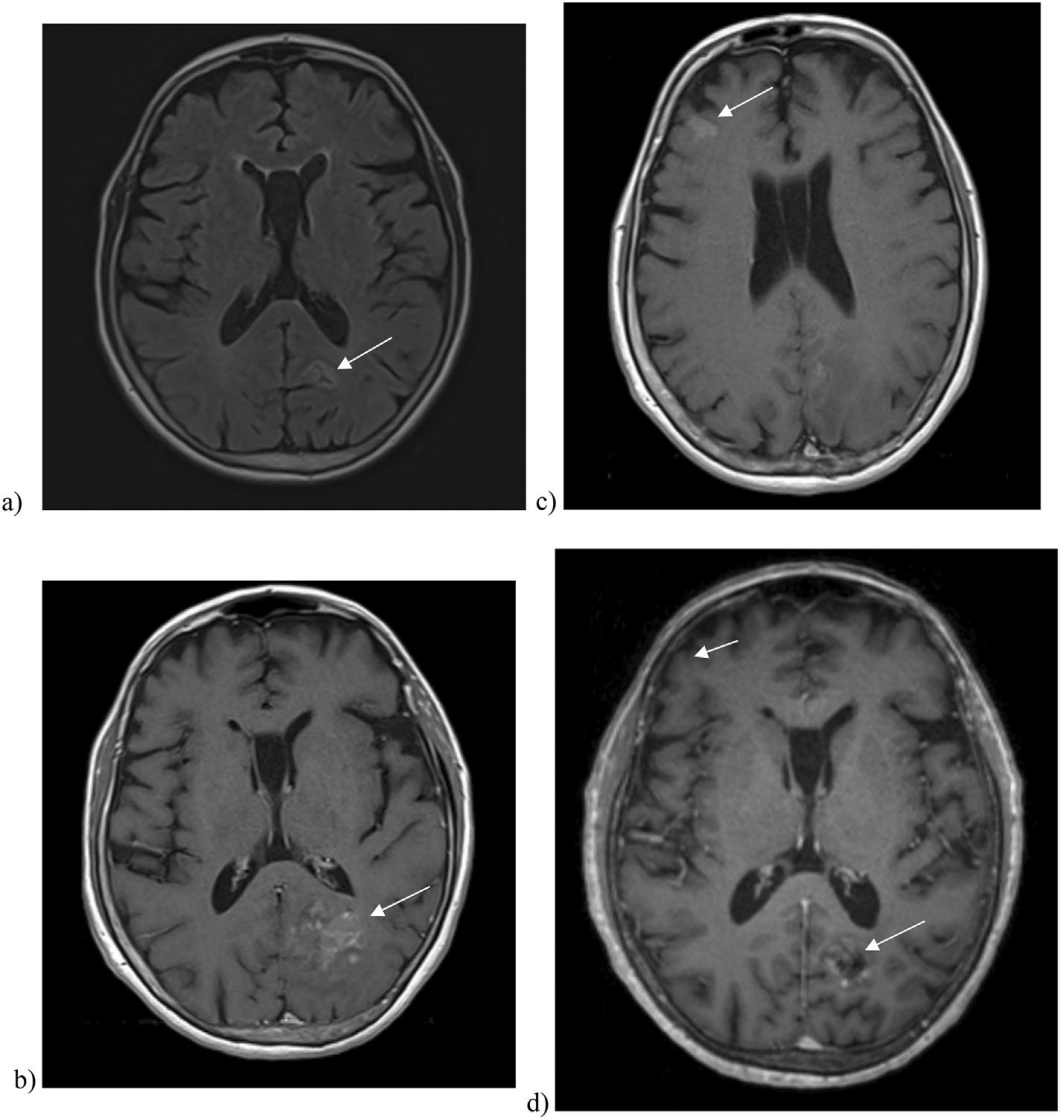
Brain MRI. (a) 1.2 cm lesion in the left inferior parietal lobe misinterpreted as a scar from an old stroke when first worked up in 2022 in axial representation. (b) Brain MRI performed 3 months after completion of an entire year of immunotherapy: a 3.3 × 2.8 cm lesion in the left inferior parietal lobe in axial representation. (c) Brain MRI performed 3 months after completion of an entire year of immunotherapy showed a small tumor measuring 1.5 cm in the right frontal lateral cortical area in axial representation. (d) Follow‐up brain MRI performed 3 months later: two brain lesions significantly decreased in size. Now measuring 2 cm versus 3 cm for the mass in the left inferior parietal lobe; and 4 × 8 mm versus 1.5 × 0.8 cm for the lesion in the right frontal lateral cortical area in axial representation. No magnification of any of the images was done. They were copied as they appeared on the viewer. The two images of the brain MRI labeled (b) and (c) were slightly modified by few millimeters in width to fit on the page.
